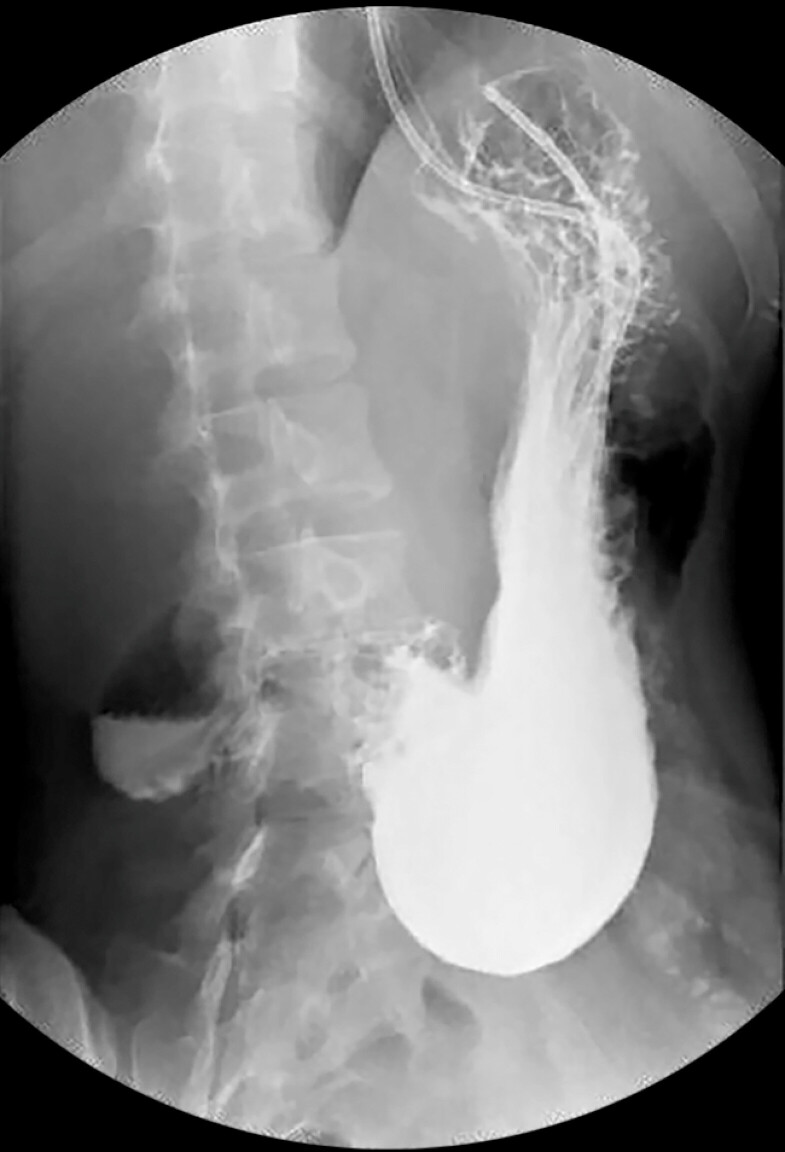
Upper gastrointestinal radiography showed a delayed passage of contrast medium through the pyloroduodenal region.
